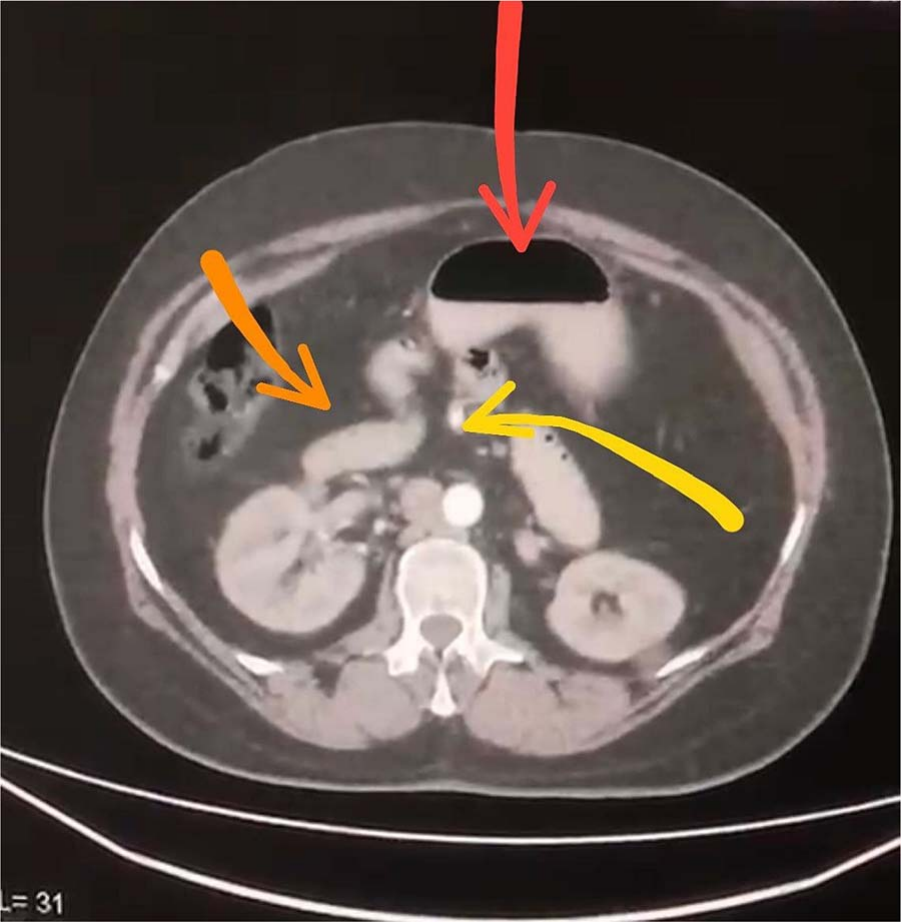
Axial contrast-enhanced CT scan demonstrating abnormal positioning of abdominal structures: The stomach is seen in the upper center (arrow), the duodenum crosses the midline anteriorly (arrow), and the superior mesenteric artery lies abnormally to the right of the superior mesenteric vein (arrow).
